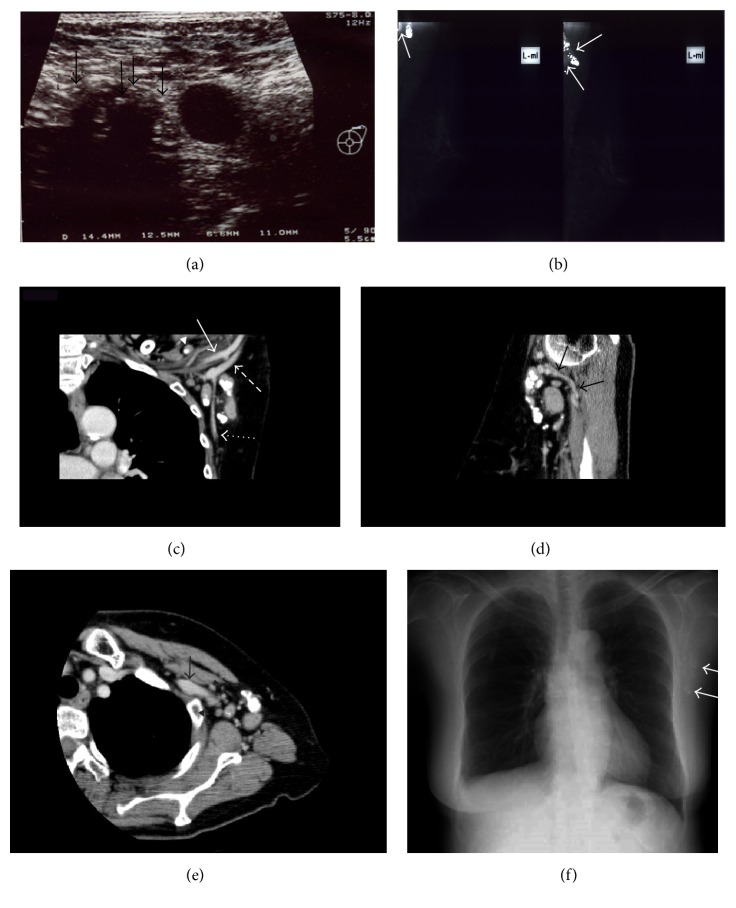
Images from a 67-year-old woman with a left axillary mass. (a) Axillary US shows well-circumscribed markedly hypoechoic ovoid lymph nodes. Every node lacks a hilum. Note the multiple rough calcifications (arrows) within the lymph node. (b) Axillary tail mammographic films show irregularly shaped macrocalcifications and matted, slightly dense homogeneous lymph nodes (arrows). Screening mammographic films show normal findings (not shown). (c) Coronal CT image after administration of intravenous contrast shows the presence of multiple well-circumscribed lymph nodes spread throughout the axillary vein (long dotted line) and dorsal thoracic vein (dotted line) in the left axilla and supraclavicular (arrowhead) and infraclavicular regions. Most nodes have large and dotted calcifications. Arrow indicates axillary artery. (d) Sagittal CT image shows the largest node, which has no calcification itself but is surrounded by nodules rich in calcification. Arrows indicate dorsal thoracic vein. (e) Axial CT image shows the lymph nodes are distributed extending from the axilla to the infraclavicular region around the axillary vein (arrow). (f) Chest radiograph shows clustered calcifications in the left deep lower axilla (arrows).
